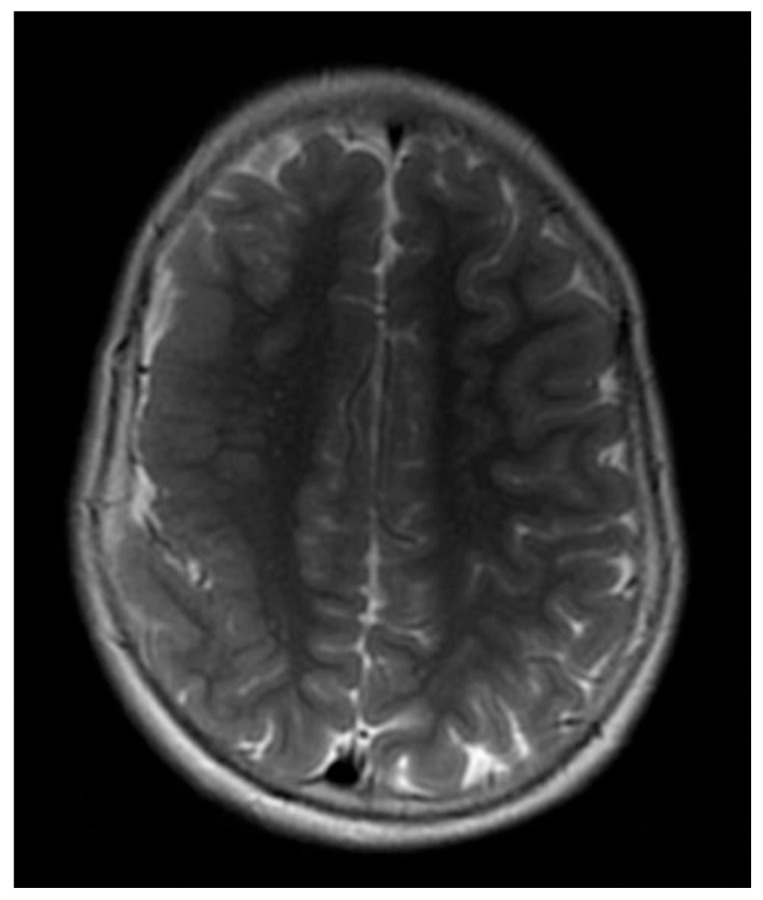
A 1.5 Tesla, T2-weighted axial scan of diffuse polymicrogyria which involves the parasagittal regions of both hemispheres and the right fronto-parietal cortex, which also appear thickened and dysplastic (Patient 13).
